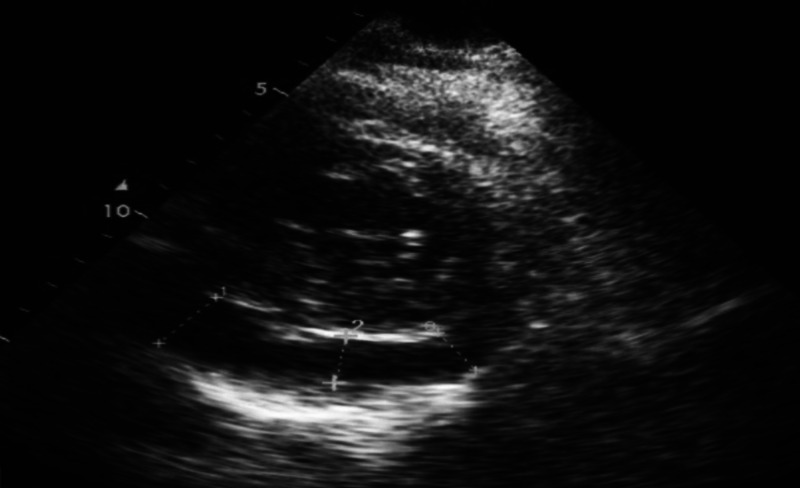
Echocardiography (parasternal view) demonstrating moderate pericardial effusion
